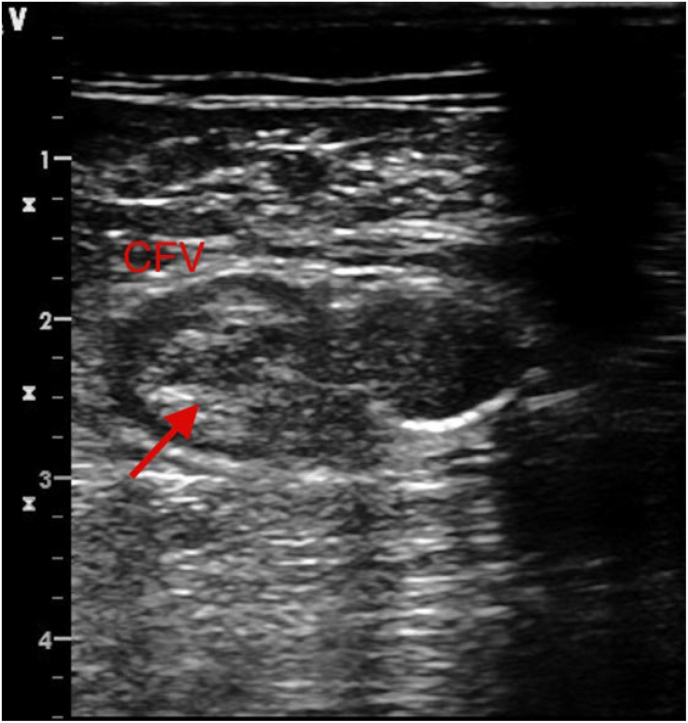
Venous Doppler ultrasound showed a thrombus in the left common femoral vein.
